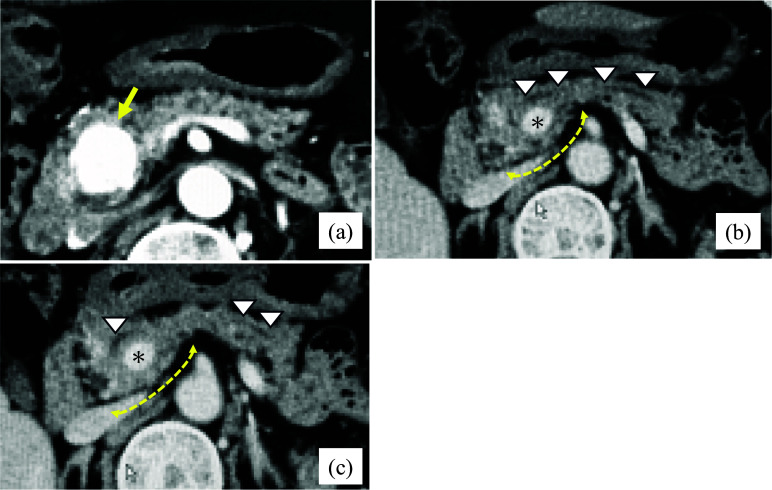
Preoperative contrast-enhanced CT; Case 1. a A 20 mm hypervascular tumor is detected in the head of the pancreas (yellow arrow). b Suprasplenic CP with anteportal MPD. c The pancreatic duct does not run in the pancreatic parenchyma on the dorsal side of the PV. Dotted yellow line: range of the dorsal parenchyma. Asterisks indicate the PV. White triangle: MPD
